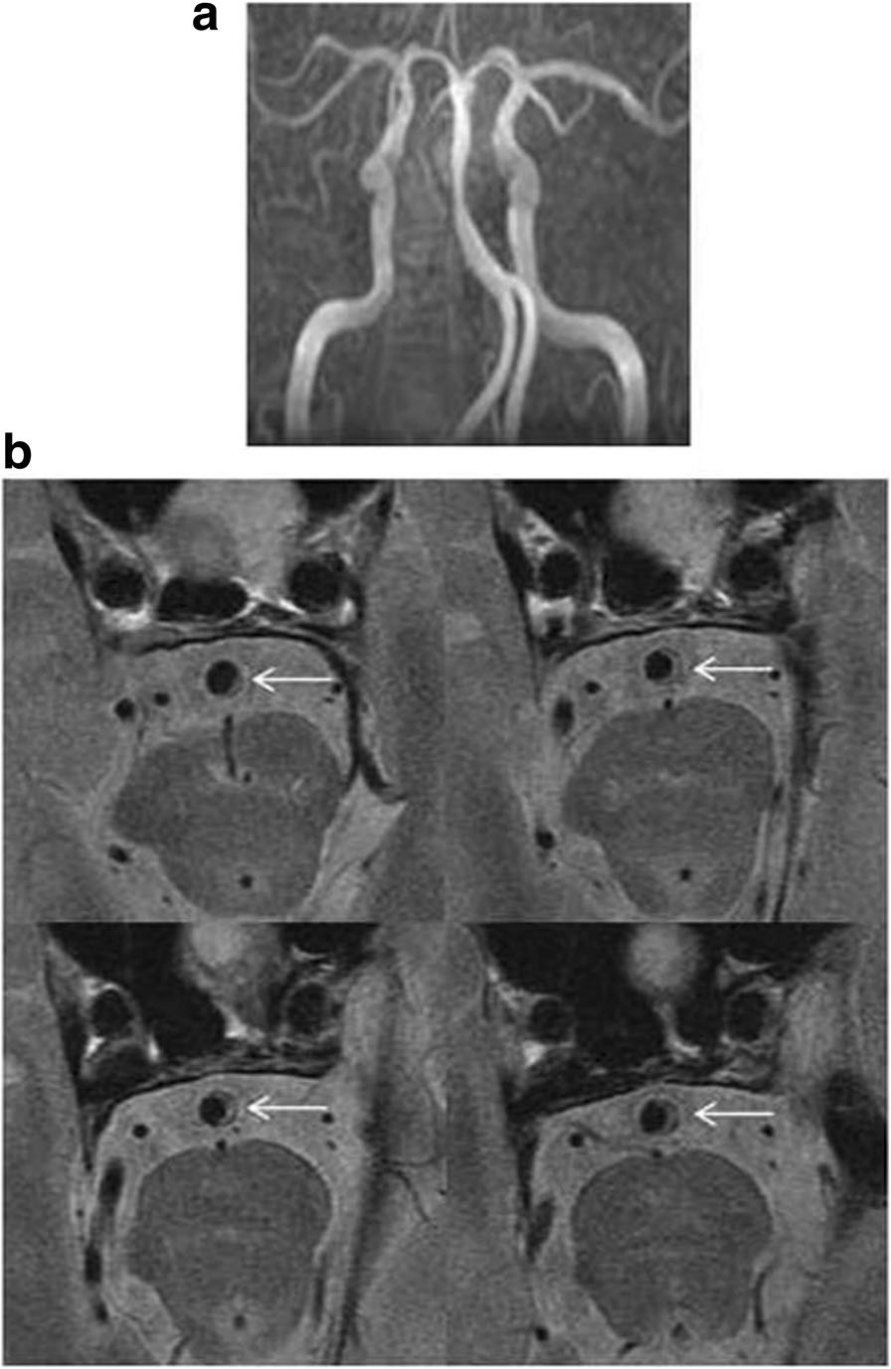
Basilar artery atherosclerosis with Walking configuration. In a basilar artery with Walking configuration (a), atherosclerosis involving lateral wall (b) can be observed on 4 of 12 consecutive slices on high-resolution magnetic resonance imaging (arrows)
